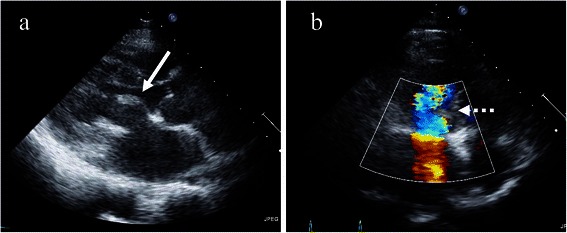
Postoperative echocardiographic images of anterior mitral leaflet (AML) plasty. (a) Long axis view. Sclerotic tissue remains in AML (white arrow). (b) Four chamber view. Mosaic pattern of transmitral blood flow near sclerotic tissue of AML on color Doppler image suggests that direction of transmitral blood flow became distorted by surging against sclerotic AML tissue (dotted arrow).
